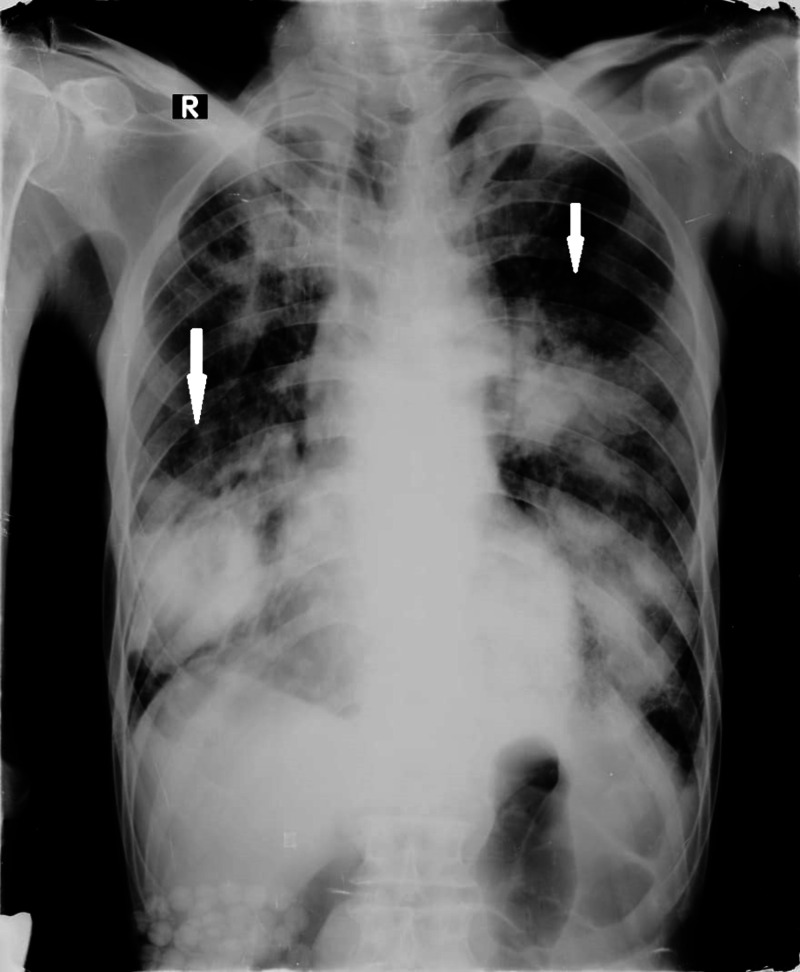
Chest x-ray This is a chest x-ray in the posteroanterior (PA) view showing bilateral infiltrates in both lower and left middle zones of lungs in the peripheral distribution
